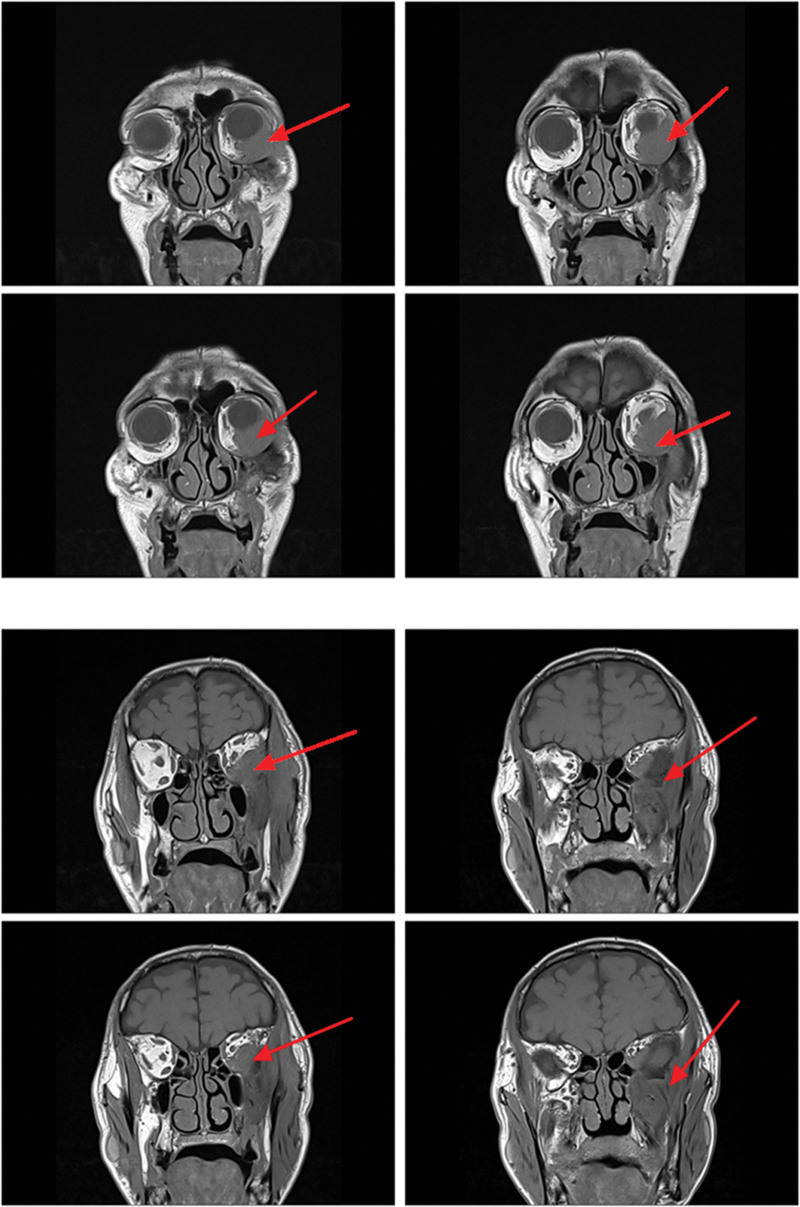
The patient MRI coronal T1 phase left posterior ocular fat space, left lateral ocular, and left inferior ocular muscular space masses are isosignal (red arrows). MRI = magnetic resonance imaging.
